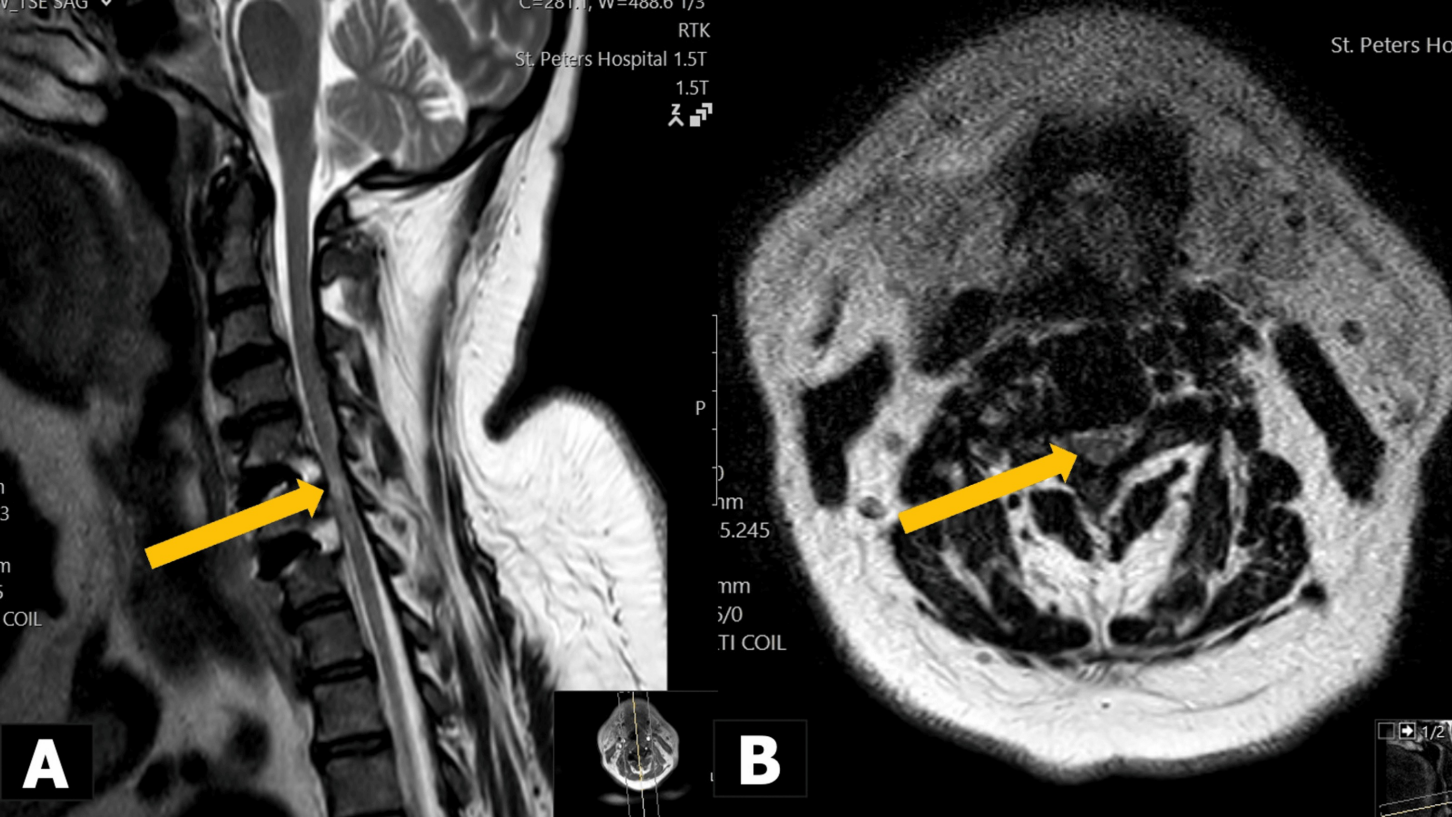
Initial postoperative T2-weighted MRI scans showing emphasis on the progressive myelomalacia at C5/C6 and C6/C7 (yellow arrows) but no recurrent cord compression in a) sagittal view and b) axial view.
